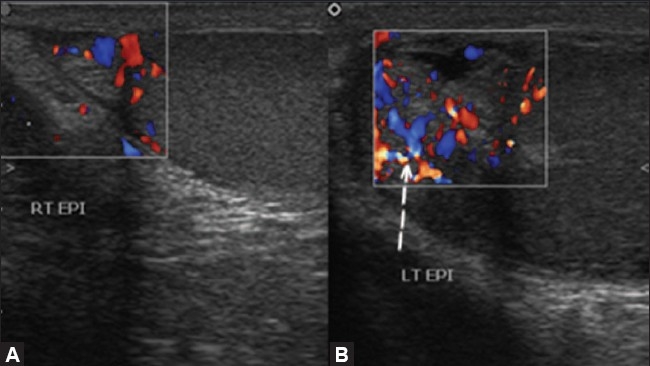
Epididymitis, Color Doppler shows an enlarged left epididymis with increased color flow (arrow in B), suggestive of epididymitis. The right epididymis is normal in size and shows normal color flow (A)
